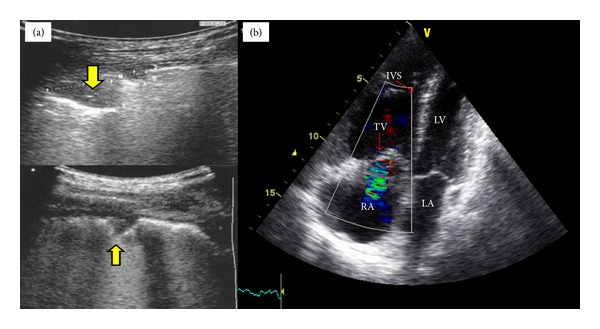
Pulmonary embolism: ((a) lung ultrasound) peripheral, triangular, and pleural based hypoechoic lesions (yellow arrows); ((b) transthoracic echo, apical view) it shows right ventricular (RV) dilation, RV hypokinesia, septal flattening, and tricuspid regurgitation. IVS: interventricular septum; TV: tricuspid valve; LV: left ventricle; RA: right atrium; LA: left atrium.
